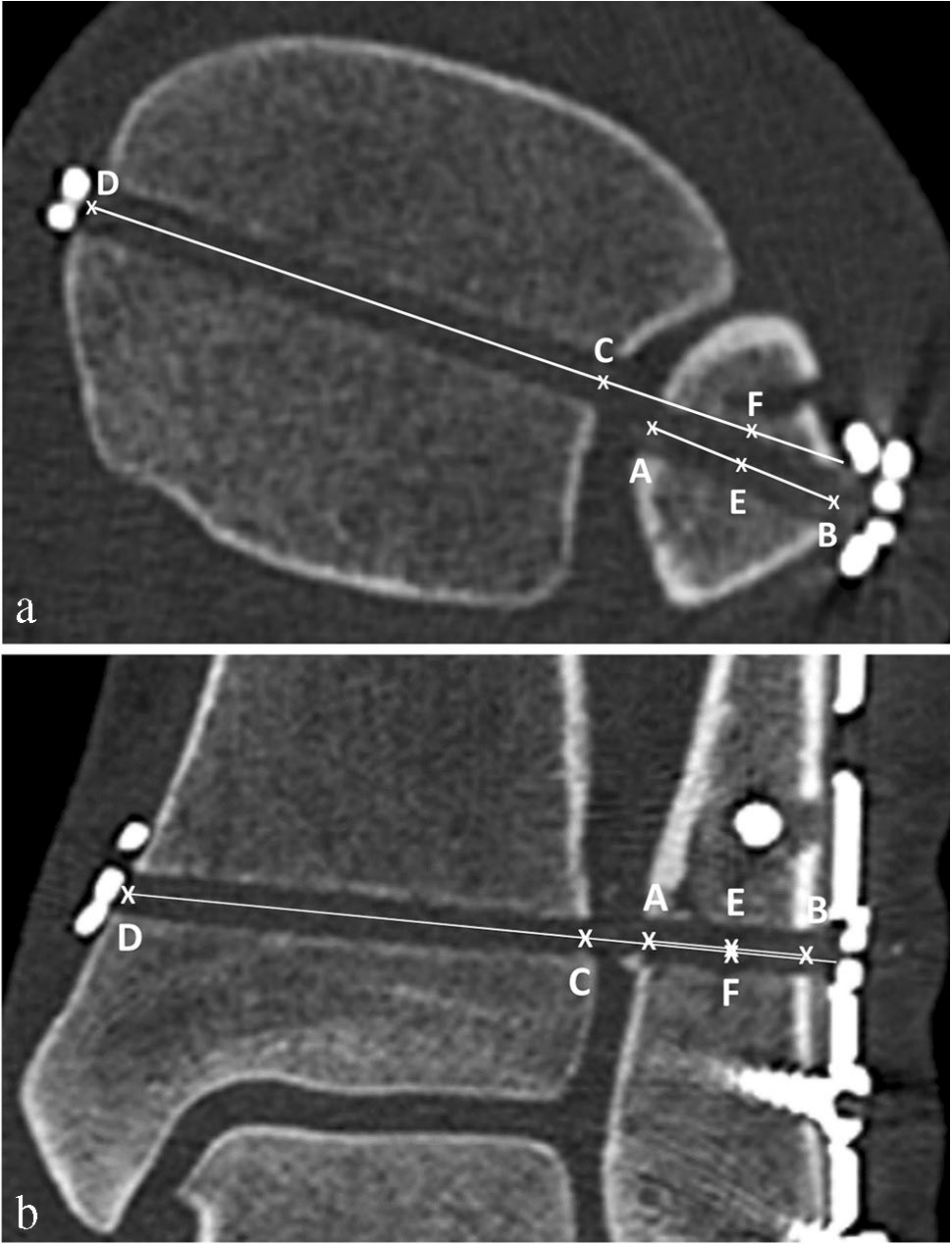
Axial (a) and coronal (b) computed tomography reconstruction of a left ankle after open reduction and internal fixation of the lateral malleolus (plate) with anatomical dynamic stabilization of the distal tibiofibular joint, lateral endobutton, and medial flip anchor. a Assessment of the axial plane: A–B fibular line, C–D tibial line, E–F transversal offset (TO). b Assessment of the coronal plane: A–B fibular line, C–D tibial line, distance E–F = vertical offset (VO)
